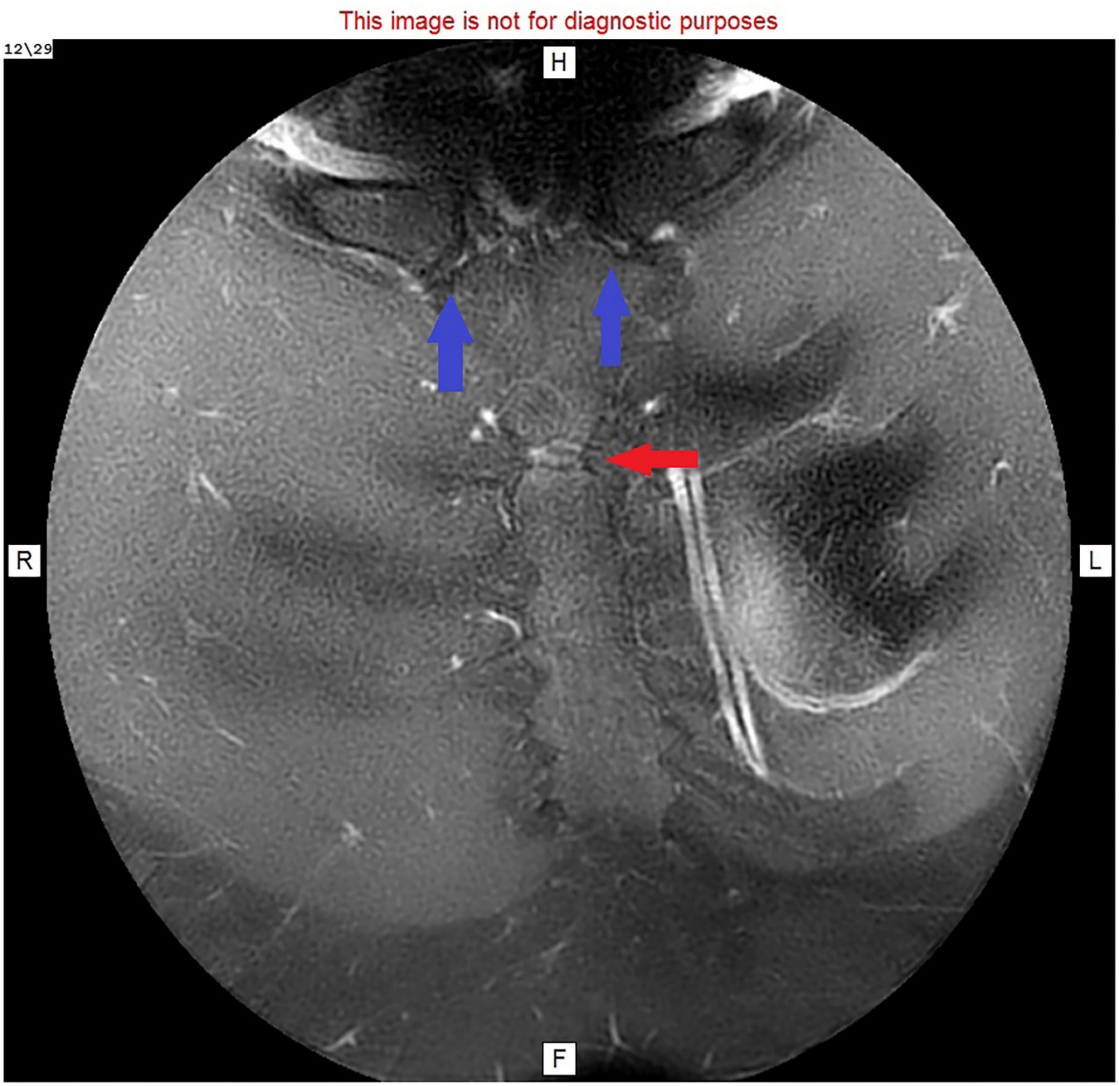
MRI image: T1 with faset and gadolinium contrast, cranial view, showing enhanced manubriosternal joint (red arrow) and normal sternoclavicular joints (blue arrows).
